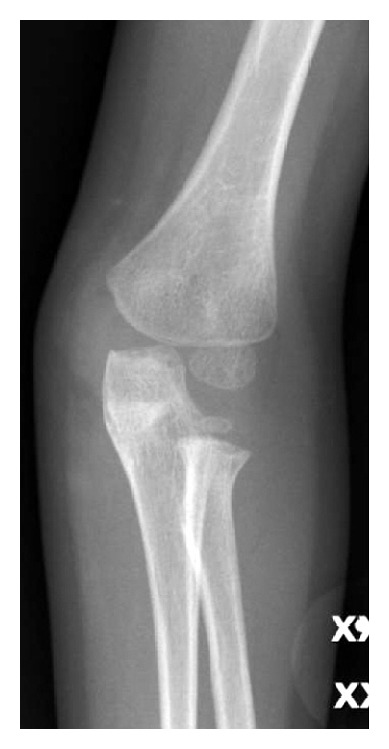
Anteroposterior radiograph four months postoperatively. Note the union of the olecranon fracture and the satisfactory position of the radiocapitellar joint.
